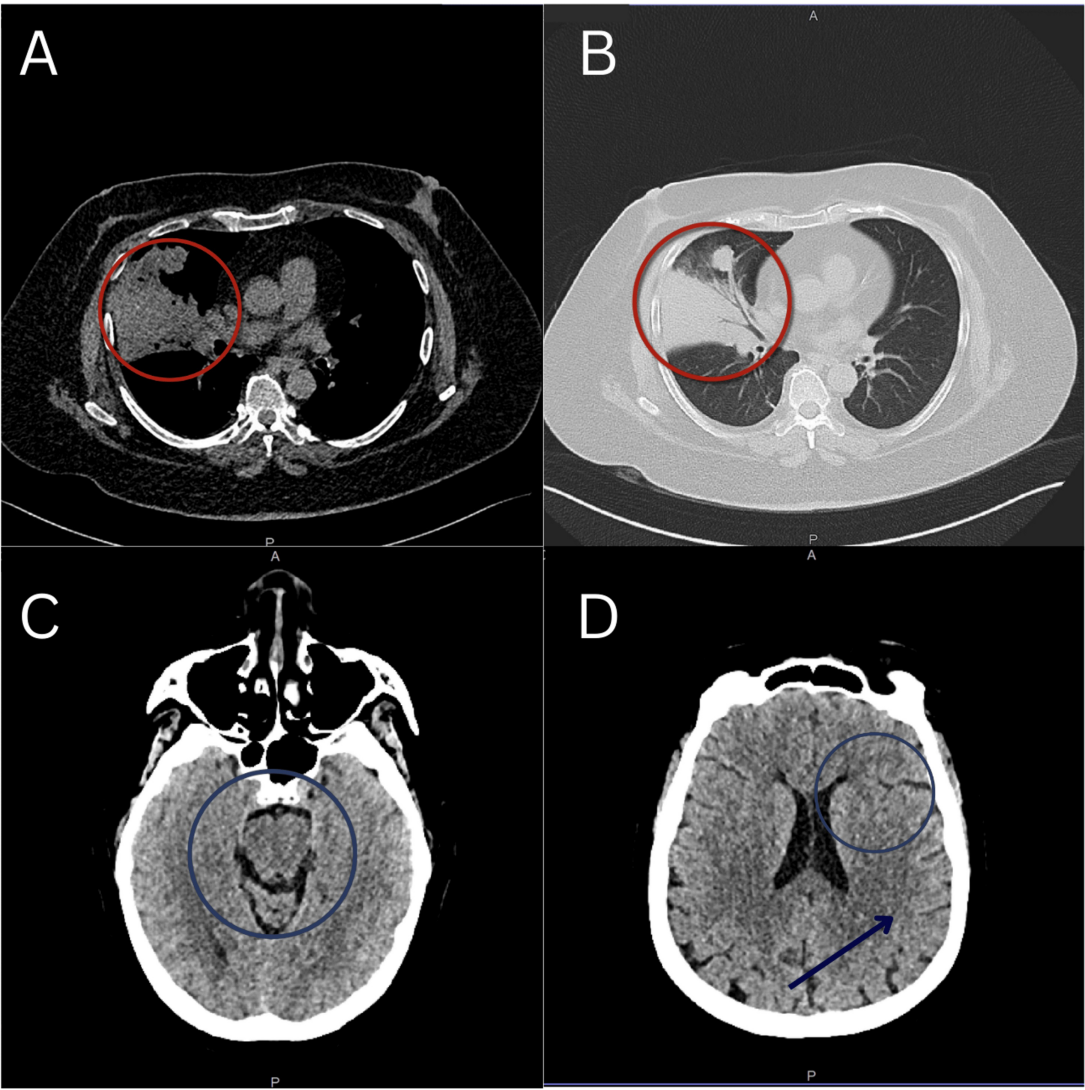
The patient's high-resolution plain chest (A and B) and cranial (C and D) CT scans. The red circles indicate the non-calcified parenchymal opacity seen in the high-resolution plain chest CT scan. The blue circle in image C shows the intact cisterns, and in image D, the intact sulci with no effacement. The blue arrow represents intact gray and white matter, indicating the absence of increased intracranial pressure.
